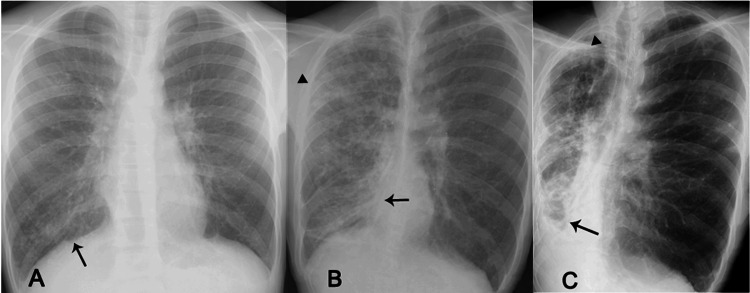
Retrospective findings on serial chest X-rays taken at the age of (A) 11 years, (B) 15 years, and (C) 25 years (A) Mild infiltration in the lower right side of the lung (black arrow); (B) Moderate infiltration in the upper (arrowhead) and volume reduction in the lower regions (black arrow) on the right side of the lung; (C) Cystic change in the apex (arrowhead)and marked bronchiectasis with tracheal shift to the right side in the lower region (black arrow) on the right side of the lung.
